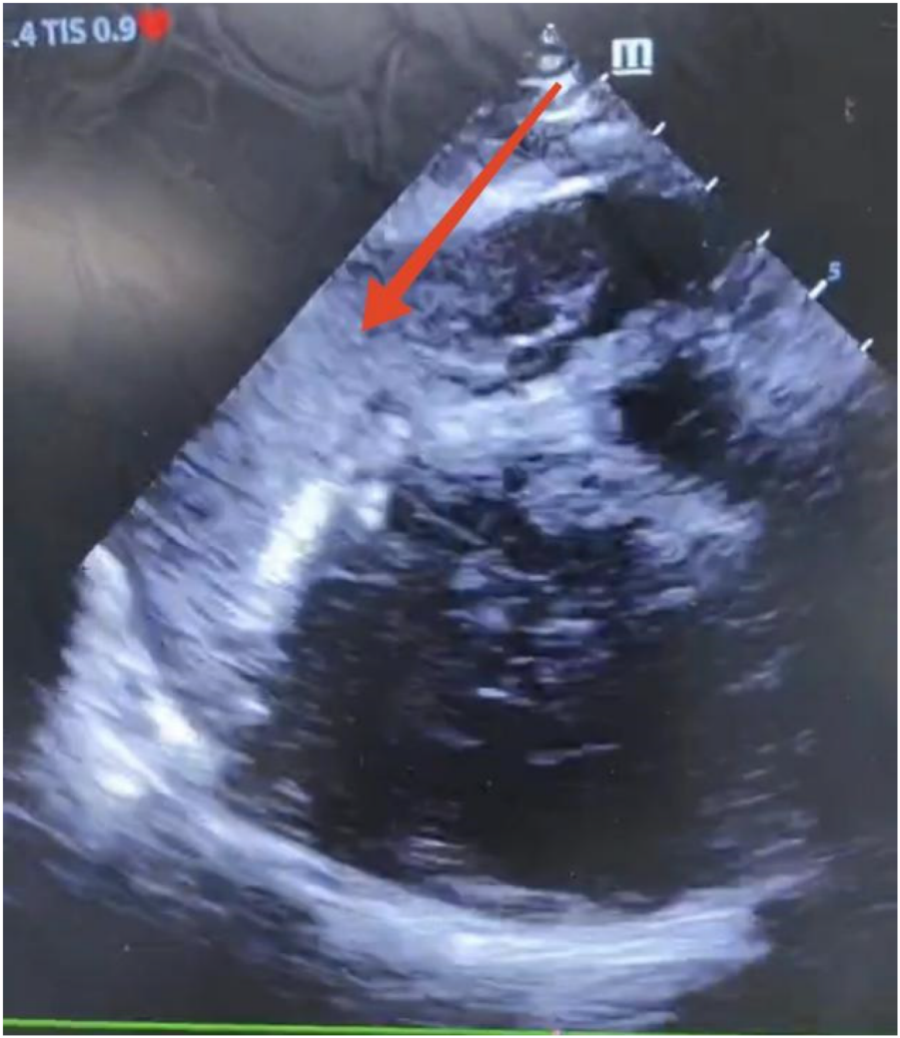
Bedside echocardiography showing a massive pericardial effusion.
